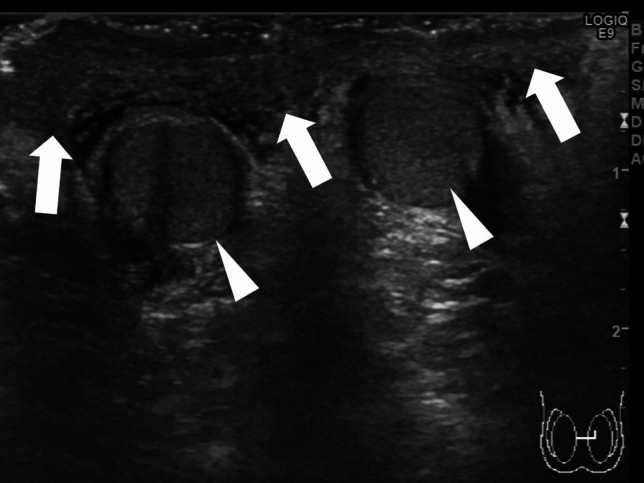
Ultrasonographic images of a 1-year-old boy with idiopathic scrotal edema. Low echoic changes due to edema in the dermis of the right and left scrota are evident. The patient was diagnosed with idiopathic scrotal edema based on these findings
